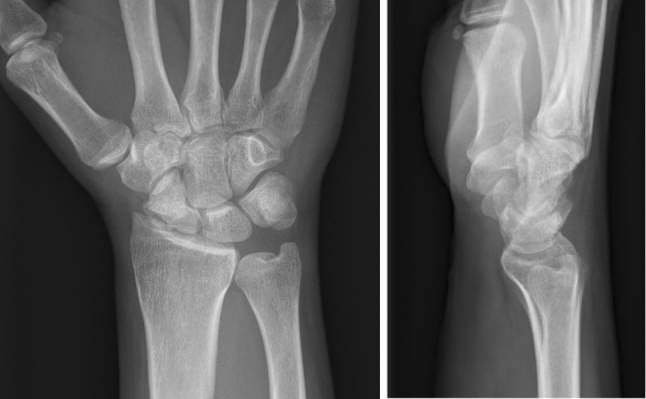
Preoperative X-rays (dorsopalmar and lateral)
